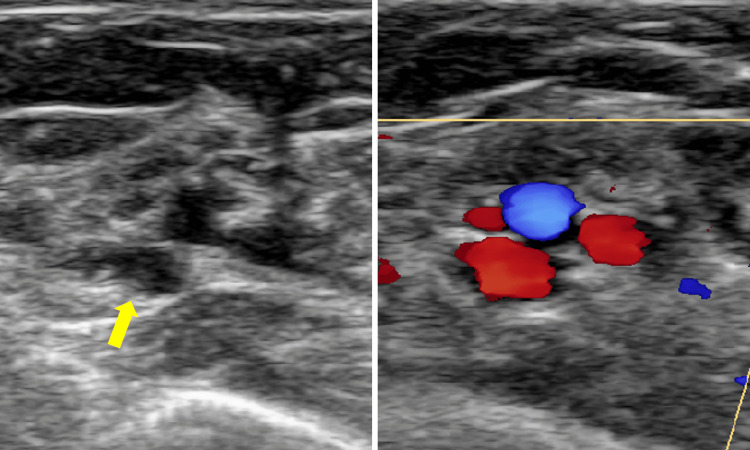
Ultrasound-guided venous evaluation The patency and course of the brachial vein were confirmed by ultrasonography, and the medial brachial vein was selected as the puncture site. Color Doppler demonstrates the veins in red and the adjacent artery in blue.
